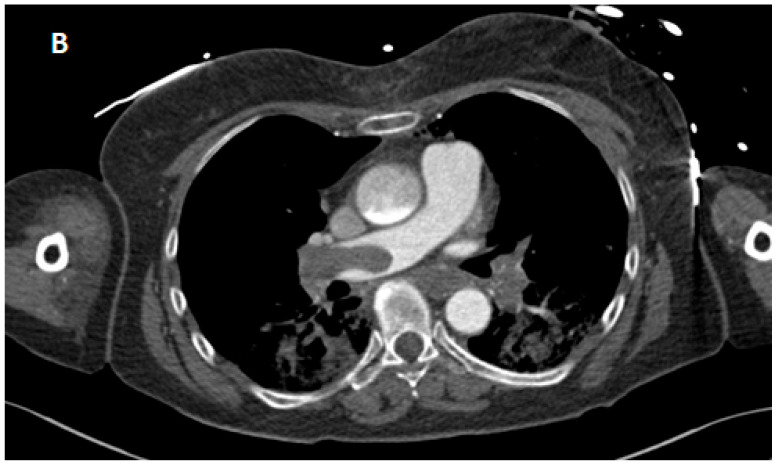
Chest computed tomography on the first day of hospitalization with evidence of central pulmonary artery embolism. (A) Lung window; (B) soft tissue window.
